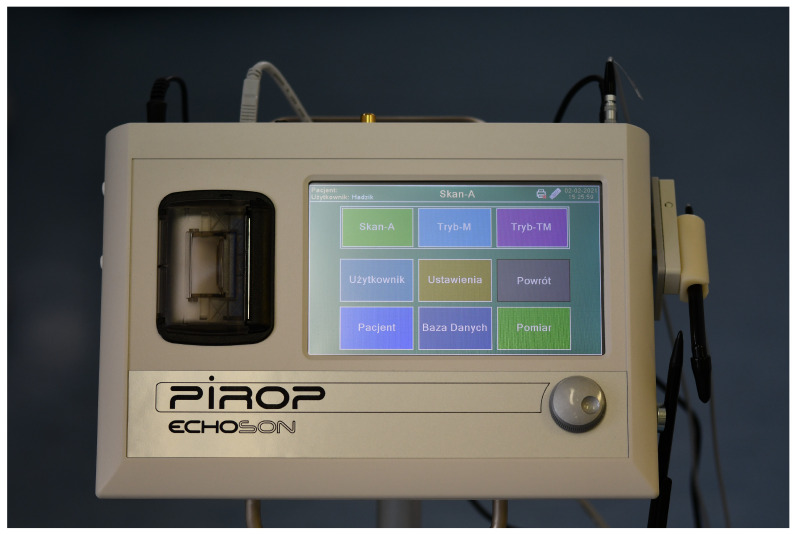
The PIROP-G ultrasound-based system (ECHOSON S.A., Puławy, Poland) used for non-invasive measurement of peri-implant soft tissue thickness in the present study.
